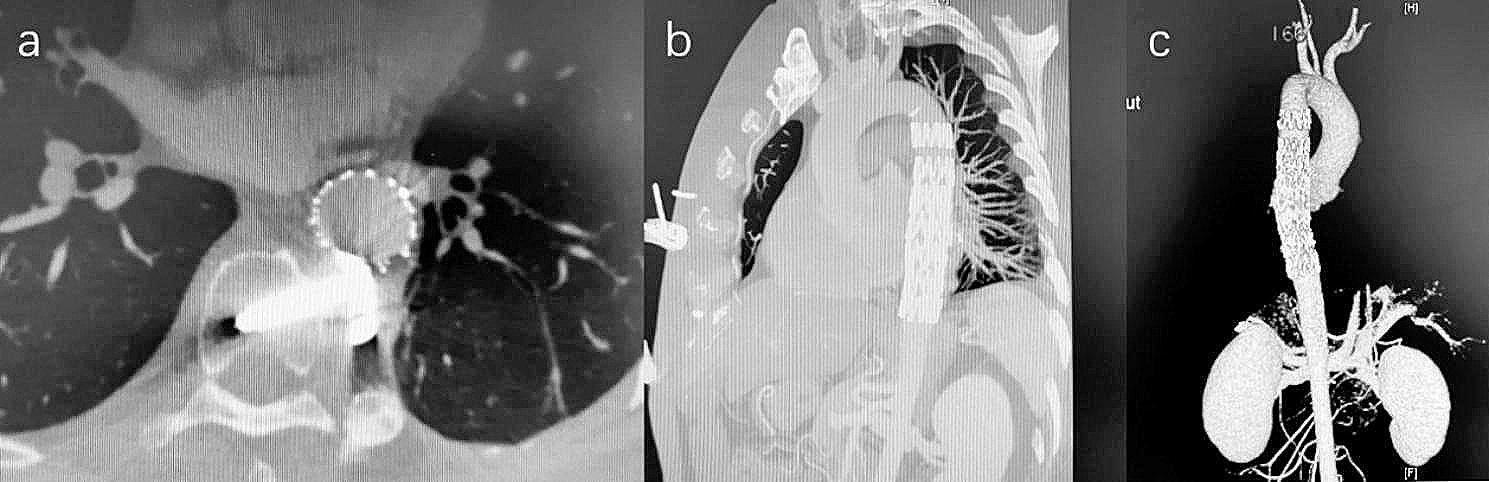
CT angiography revealed complete exclusion of the pseudoaneurysm with no endoleak
